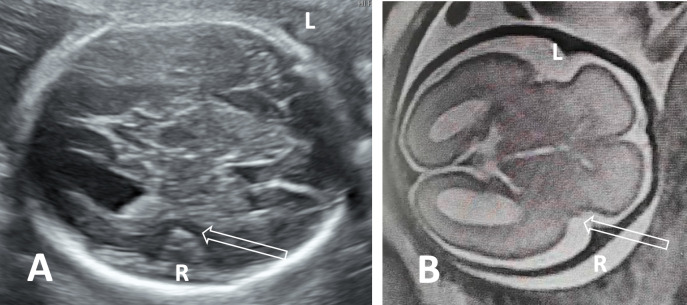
Patient 31, the 27.1-gestational-week-old fetus of a 29-year-old woman, had a narrow plateau-like insula in the right hemisphere. A Axial plane shows the comparison of the abnormal SF (hollow arrow, R) and the normal SF (L); B axial plane by MRI shows similar characteristics of SF (hollow arrow, R) with ultrasound. WES-Trio revealing a variant in the *CYNC1HI* gene that may cause mental delay
